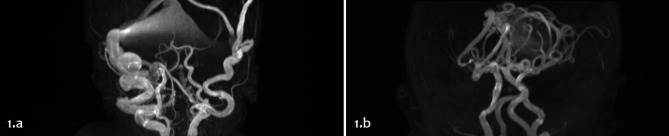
Brain MRI findings of intracranial AVSs in sagittal planes, revealing a DAVF of the posterior cranial fossa in patient 1 (1.a) and a VGAM in patient 2 (1.b)
